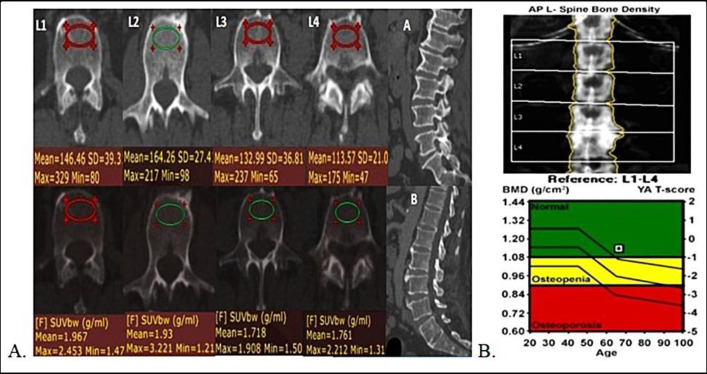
A. Initial staging axial PET/CT images in a 67 year old female with IDC breast carcinoma in top row show reduced VHU with degenerative osteophytes (L1-L4 mean HU of 139). Fused axial PET/CT images in bottom row show SUV_max_ values of L1-L4 vertebrae. However no fractures were seen. Sagittal CECT images (A and B) also show marked degenerative changes in the spine. B. DXA scan results of this patient show mean L1-L4 T- scores -0.4, above the cutoff limit of < -1.0 used to define osteopenia on DXA scans. This is a case of false negative DXA scan overestimating T - scores because of degenerative changes
